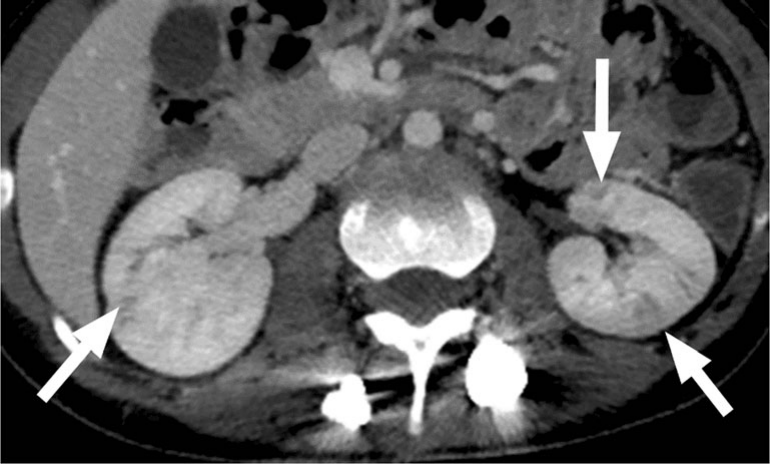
Pyelonephritis: a 26-year-old paraplegic female who presented with diffuse abdominal pain. Axial image from contrast-enhanced CT shows striated enhancement of the kidneys bilaterally, with white arrows indicating linear regions of decreased enhancement
